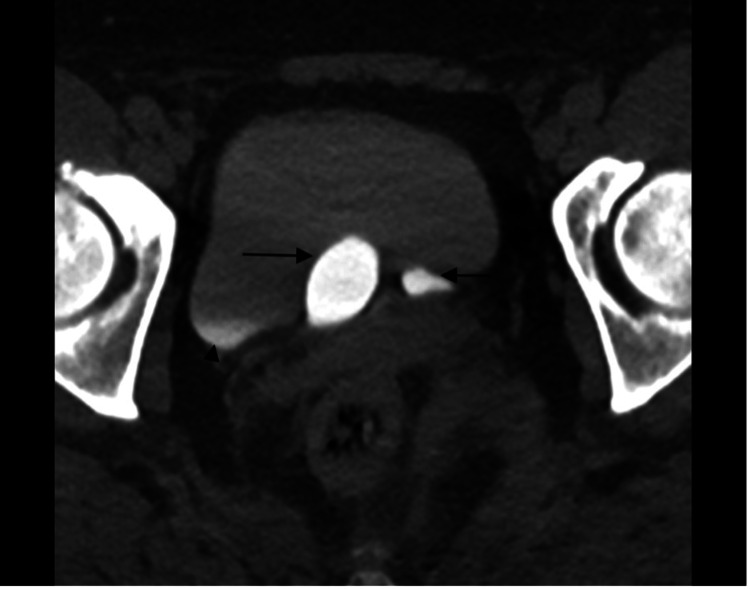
Axial IV contrast-enhanced CT image through the pelvis in the delayed urographic phase shows two well-circumscribed structures at the bladder trigone (arrows) which are opacified with contrast. A small amount of excreted contrast is also seen in the dependent portion of the urinary bladder lumen (arrowhead).
